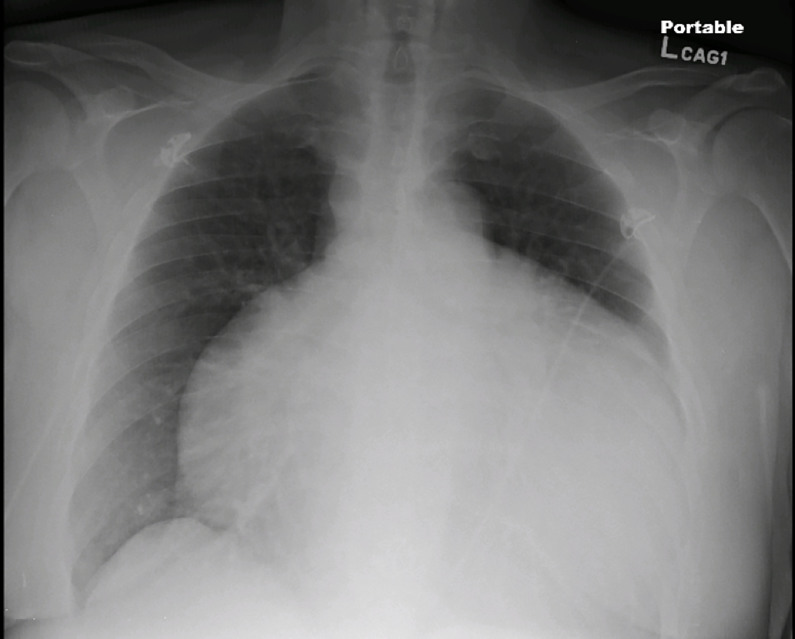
Anterior-posterior chest radiograph demonstrating massively enlarged cardiac silhouette suggestive of a large pericardial effusion
